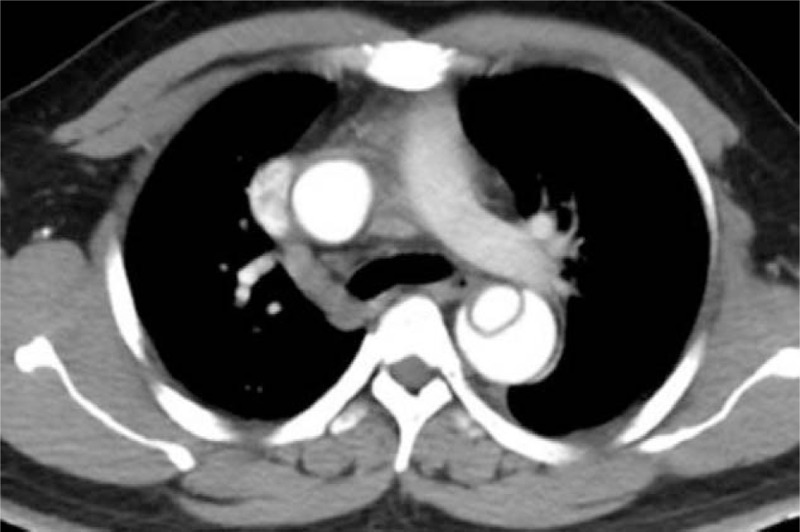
A 31-year-old male patient. The true and false lumens are revealed in these contrast-enhanced CT angiography images. CT = computed tomography.
